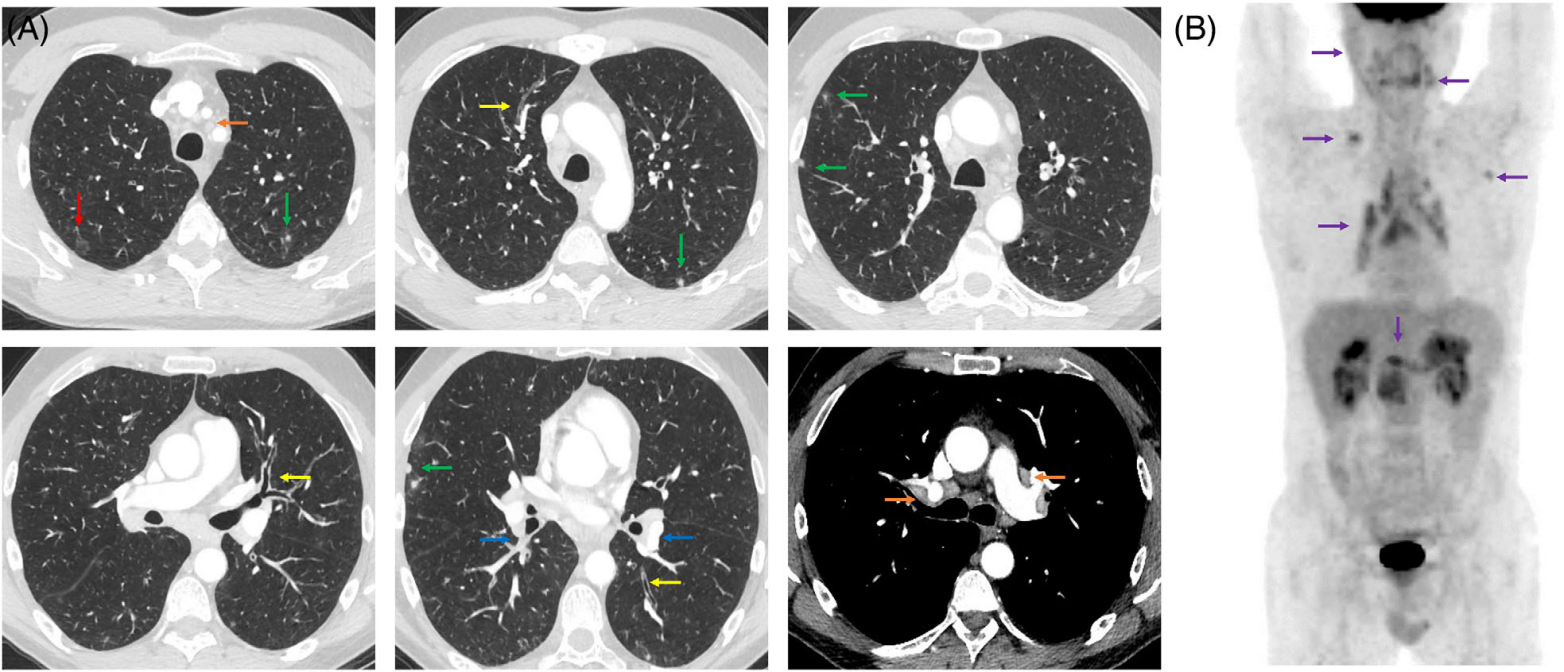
(A) Chest CT‐scan at diagnosis: multiple and heterogeneous pulmonary lesions of IgG4‐RD: Red arrow: ground glass area; Orange arrow: lymphadenopathies; Green arrows: nodules mainly sub‐pleural; Yellow arrows: bronchial wall thickening; Blue arrows: peribronchovascular thickening. (B) Body PET‐scan before Rituximab. Purple arrows: Increased ^18^FDG uptake (PET scan) of salivary glands, mediastinum, pulmonary nodules and pancreas
